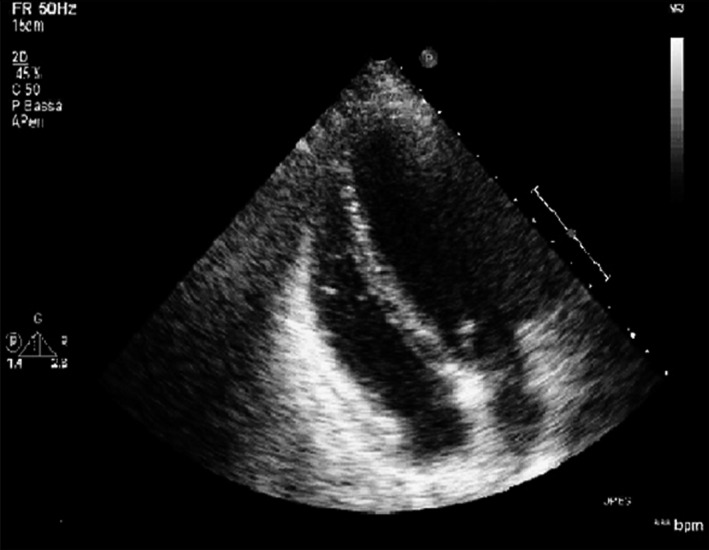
Transthoracic (TT) echocardiogram showing diffuse transmural hyperechoic myocardium and small circumferential pericardial detachment with hyperechoic aspect of the pericardium more evident on right sections.
